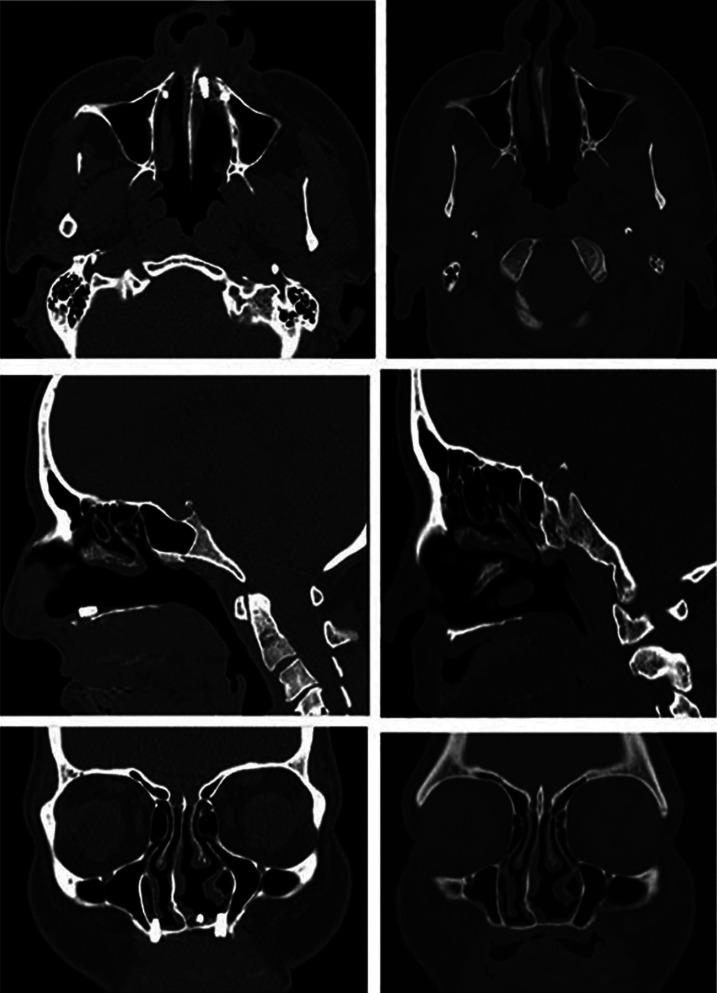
Axial, sagittal and coronal CT scan slices before and after spontaneous expulsion through the nose.
